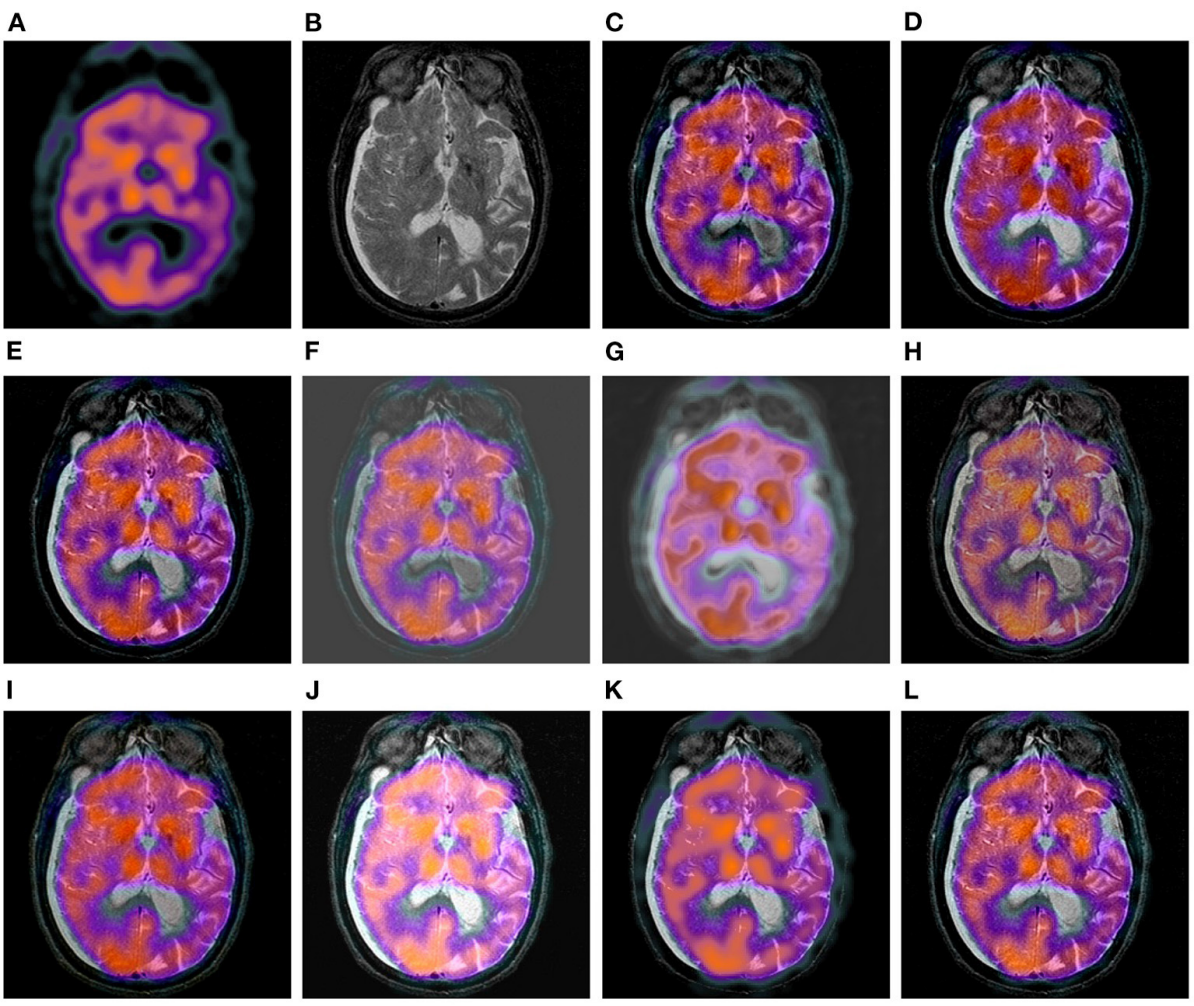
Comparison example on the SPECT-MR image dataset. (A,B) are the original SPECT image and MR image, respectively. (C–L) are the fusion results of DWT, GFF, LPSR, U2Fusion, DDcGAN, IFCNN, EMFusion, DRBIF, LEGFF_0_, and our method, respectively.
